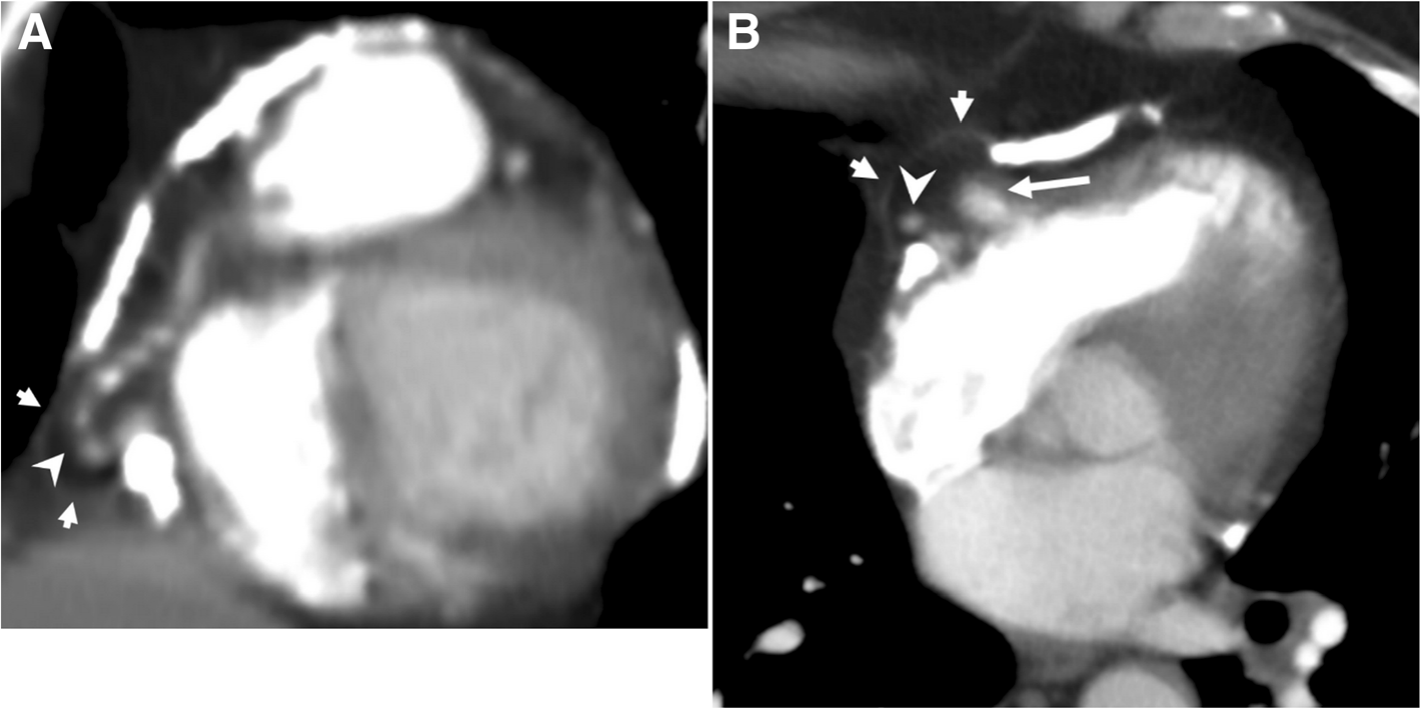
62-year-old woman with echocardiography findings suggestive of restrictive cardiomyopathy or constrictive pericarditis. Contrast-enhanced CT images on reformatted coronal (a) and axial plane (b) demonstrate linear pattern of extensive pericardial calcification. A pericardial diverticulum (short arrows) containing epicardial fat, coronary artery (arrowheads) and lateral wall of the right ventricle (long arrow) is noted
